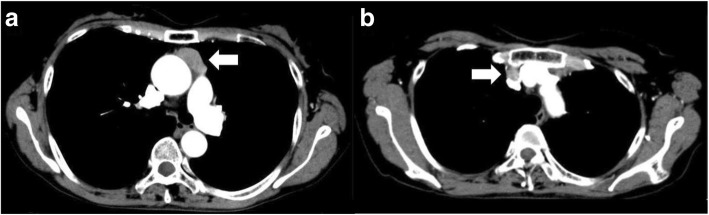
Chest CT image. A tumor (30 × 30 × 14 mm) without invasion localized in the anterior mediastinum (a). Enlargement of lymph node (b)
